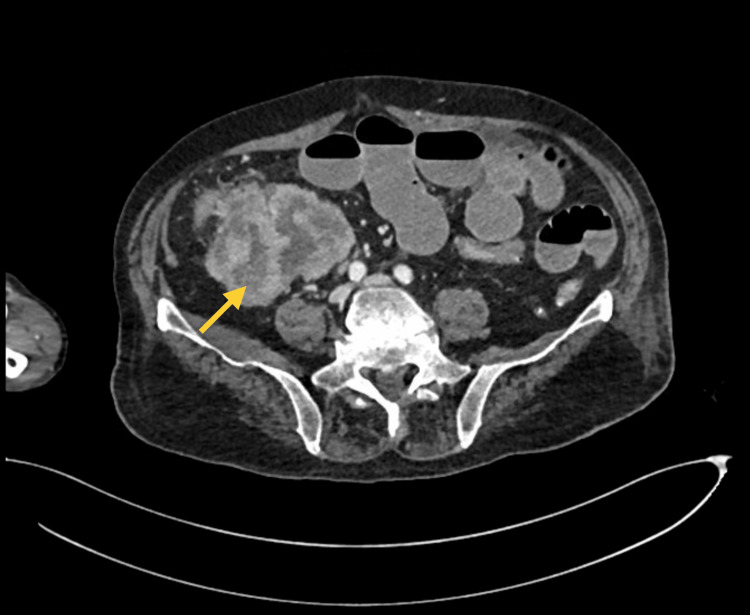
CT scan with contrast showing the mass in the ileocecal junction (yellow arrow) CT: computed tomography
